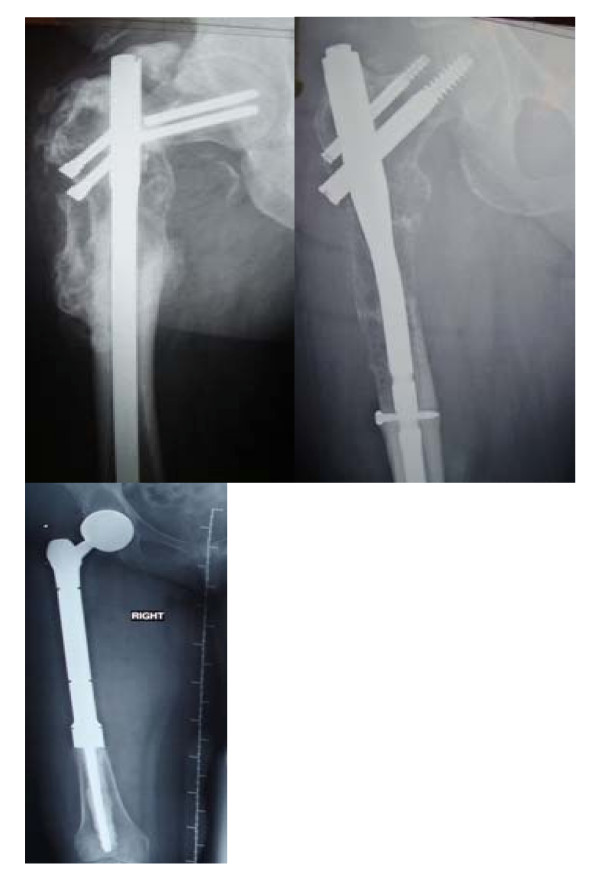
Typical indications for a proximal femoral replacement. a) Failed fixation of a proximal femoral fracture due to metastatic breast carcinoma b) Progressive destruction of proximal femur by metastatic renal carcinoma c) Radiograph of the modular endoprosthetic replacement at 12 months.
